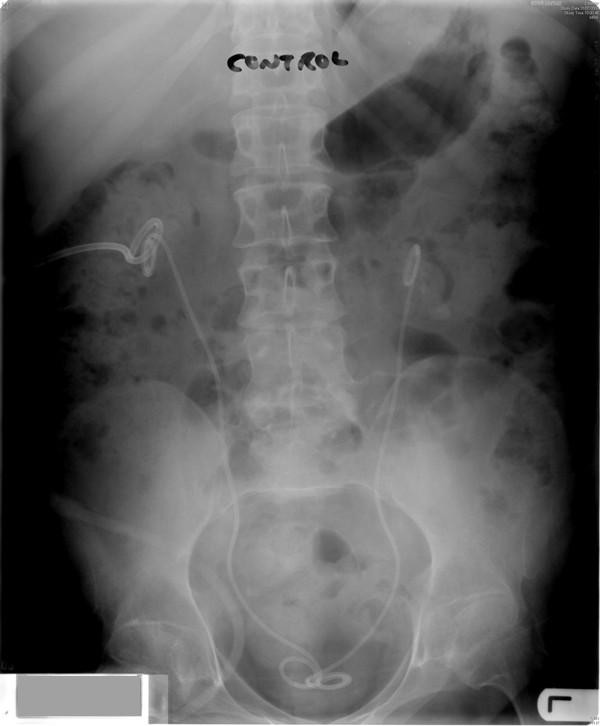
X-ray of abdomen (31 July 2006) showed stents in both ureters. Nephrostomy catheter was seen in right kidney. Left nephrostomy had been removed.
